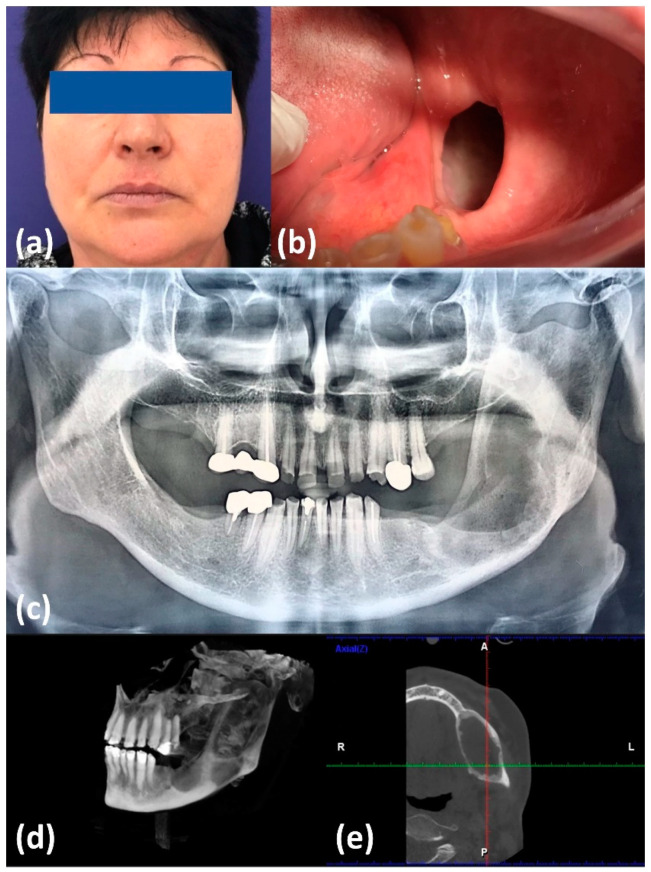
Clinical and imagistic aspect at 3 months after marsupialization. (a) Extraoral aspect; (b) intraoral aspect; (c) panoramic X-ray; (d) CBCT with 3D reconstruction; (e) CBCT axial view.
